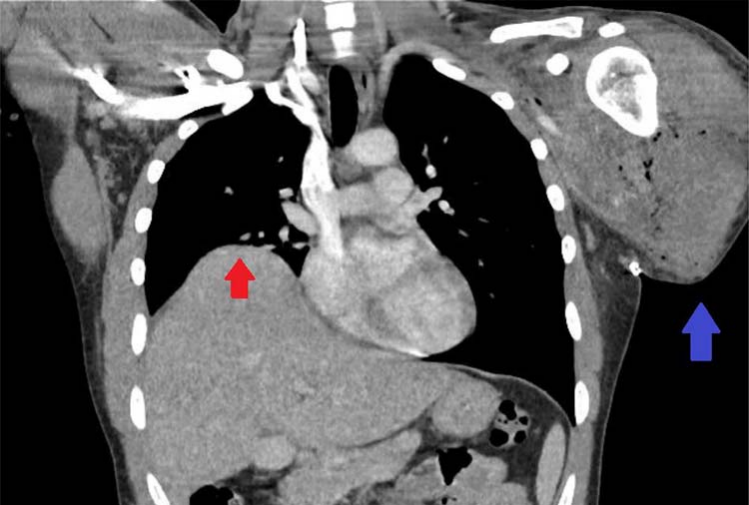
CT scan of the chest showing right-sided elevation/eventration of the diaphragm concerning for phrenic nerve injury (Red arrow); post-operative changes to LUE stump (Blue arrow).
